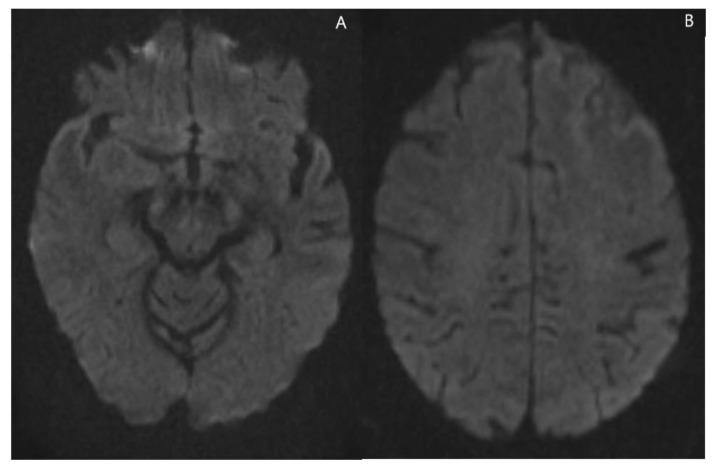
(A,B) Axial diffusion-weighted MRI demonstrating no restricted diffusion of the dominant right temporal and left frontal lesions. The other lesions (not shown) demonstrated no restricted diffusion.
